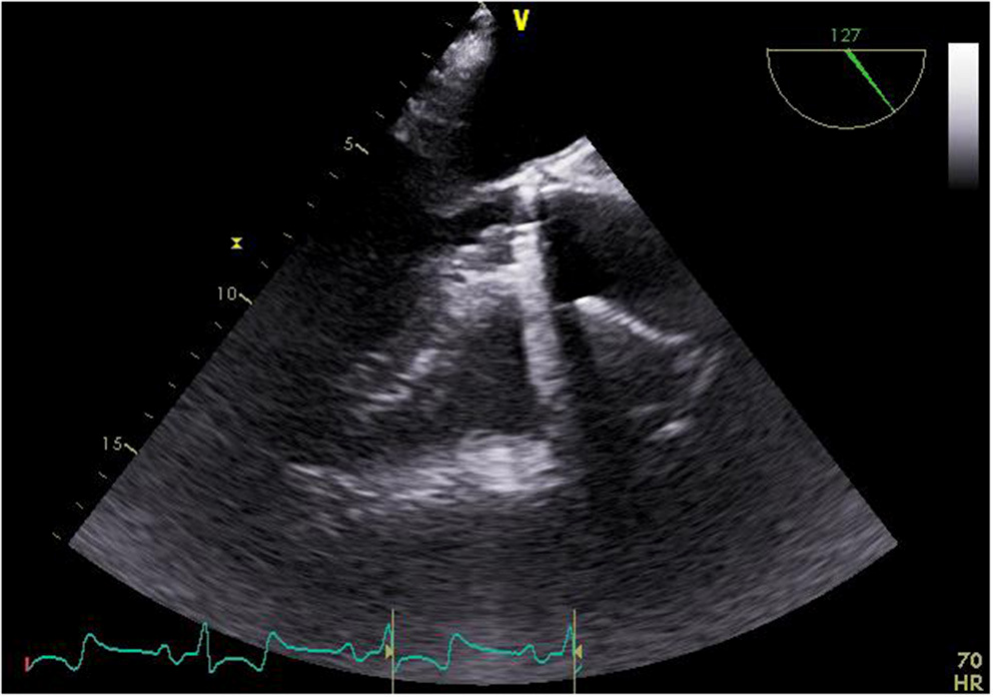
Echocardiographic finding of the vegetation at the annulus of the implanted valve prosthesis.
